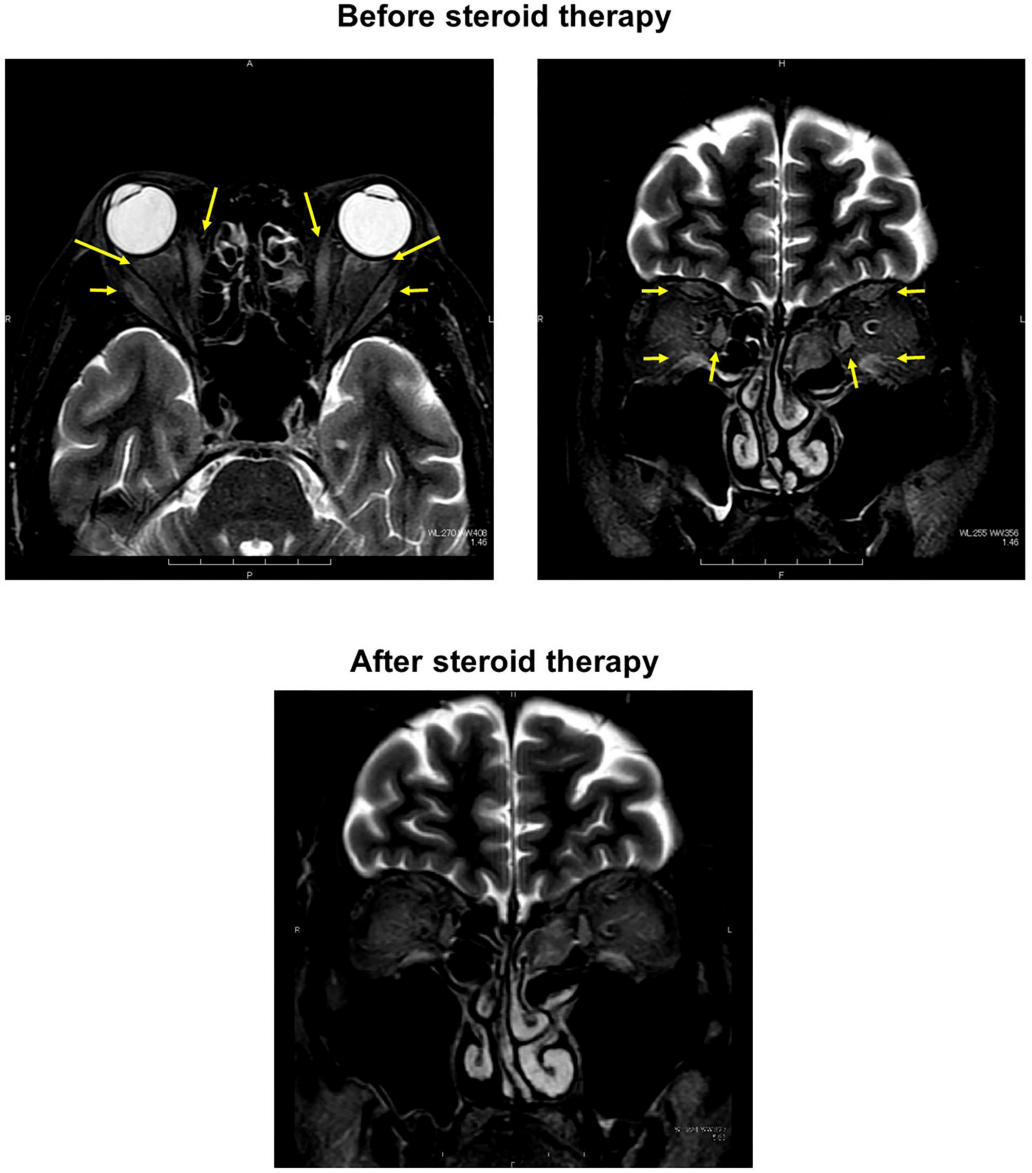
(Upper) In orbital magnetic resonance imaging before starting treatment, some high signal was detected in both extraocular muscles, indicating that inflammation extended into the extraocular muscles in this subject (both panels; yellow arrows). (Lower) After the steroid pulse therapy, signal intensity in extraocular muscle tended to be weakened, and orbital fat mass was moderately reduced.
